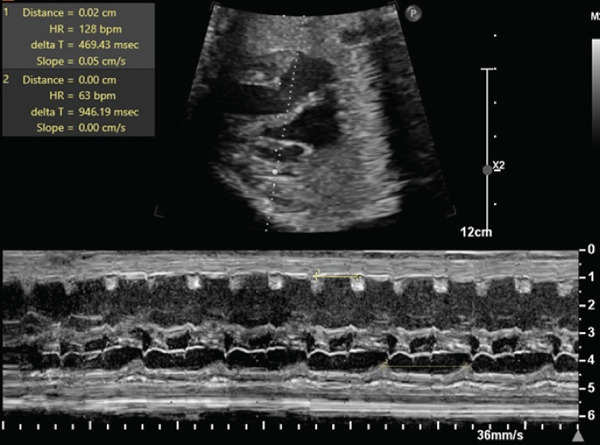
Fetal echocardiogram M‐mode image for Case 1 depicting 2:1 AV block with ventricular rate 63 bpm and atrial rate 128 bpm.
